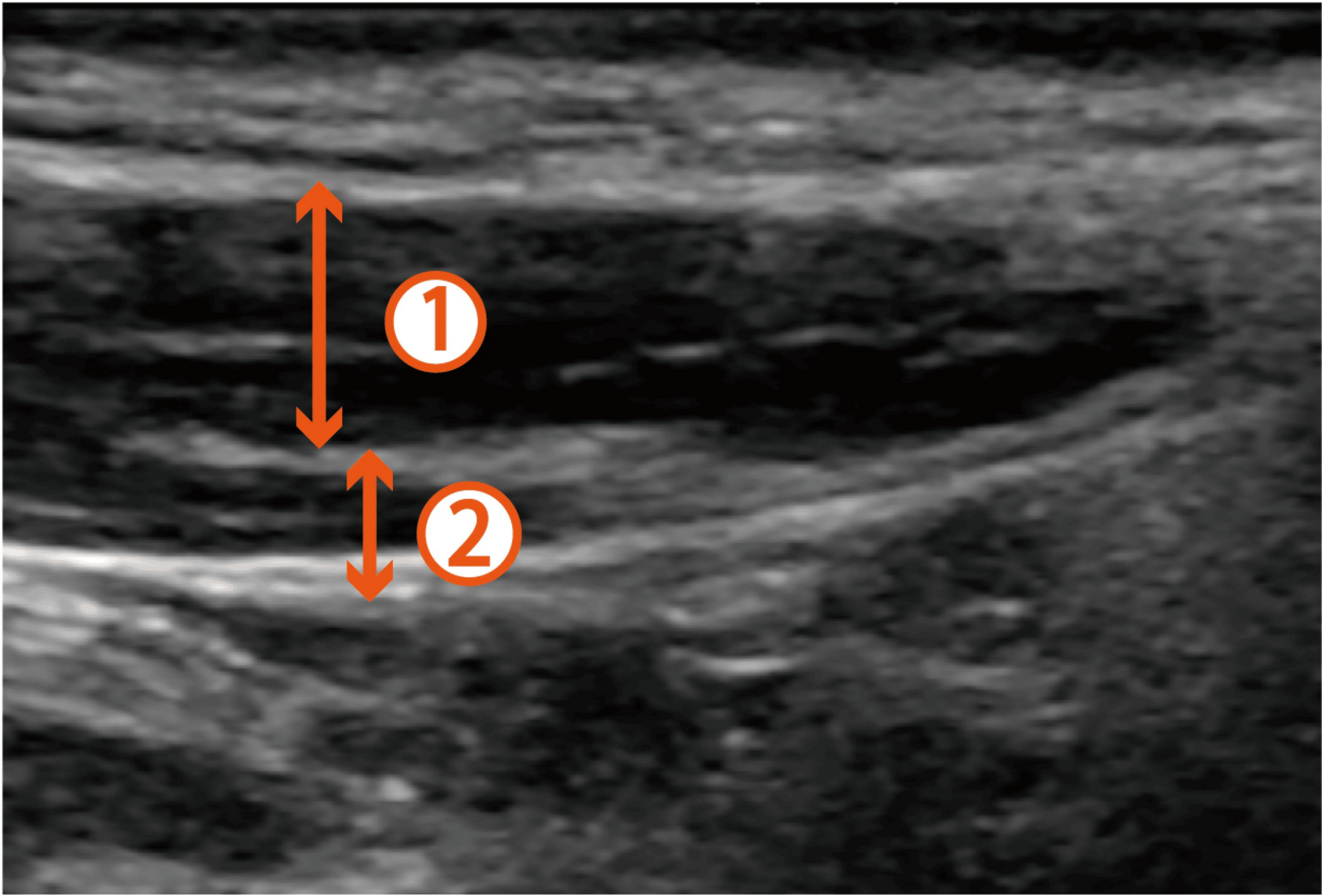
Ultrasound-guided selective glossopharyngeal nerve block in Case 1. After identification of the sternocleidomastoid muscle, a linear ultrasound probe was set parallel to the sternocleidomastoid muscle. 1: Sternocleidomastoid muscle; 2: Stylohyoid muscle.
